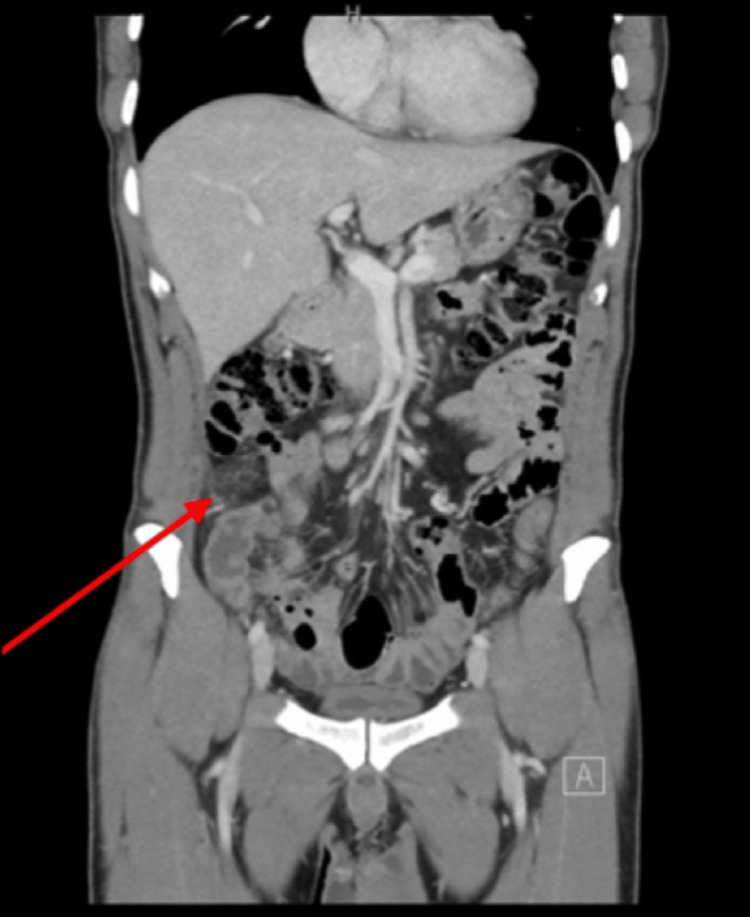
Contrast-enhanced CT of the abdomen and pelvis demonstrating primary OI Coronal contrast-enhanced CT image showing a focal area of heterogeneous fat stranding and inflammatory changes in the right lower quadrant within the greater omentum (red arrow), with no evidence of appendiceal inflammation or other intra-abdominal pathology. OI, omental infarction
